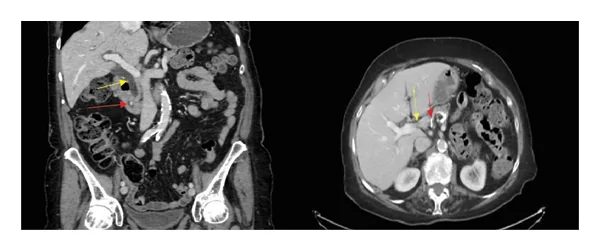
Computed tomography (coronal and axial views) on admission showing a clip obstructing the distal common bile duct (red arrows) and interval worsening of dilation of the common bile duct (up to 15 mm) and intrahepatic biliary ducts (yellow arrows).
